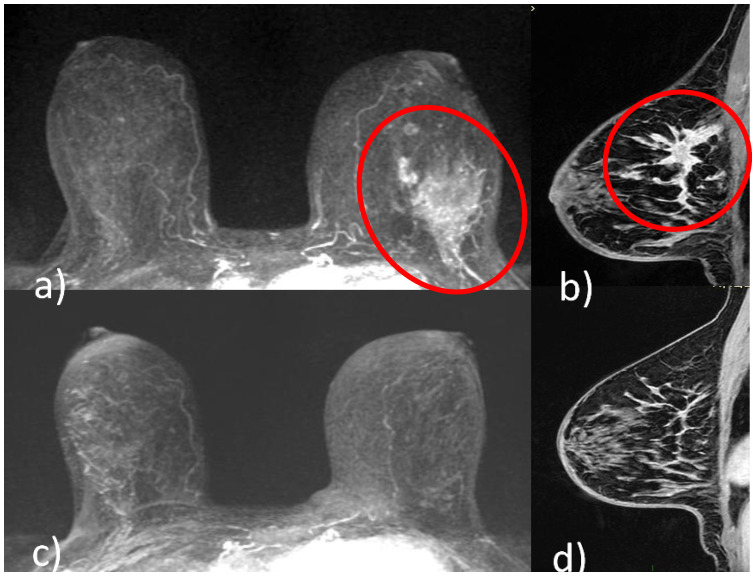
A 51-year-old patient with invasive ductal carcinoma (ER 40% PR 3% Ki67 40% HER2 3+) of the upper outer quadrant of the left breast. Pre-NACT CE-MRI revealed an area of non-mass enhancement with segmental distribution in the left breast that extends to pectoralis muscle without signs of invasion (red circle, (a) axial maximum intensity projection reconstruction image; red circle, (b), sagittal post-contrast T1-weighted image). At the end of NACT, CE-MRI showed no residual tumor (pCR) ((c) axial maximum intensity projection reconstruction image; (d) sagittal post-contrast T1-weighted image).
